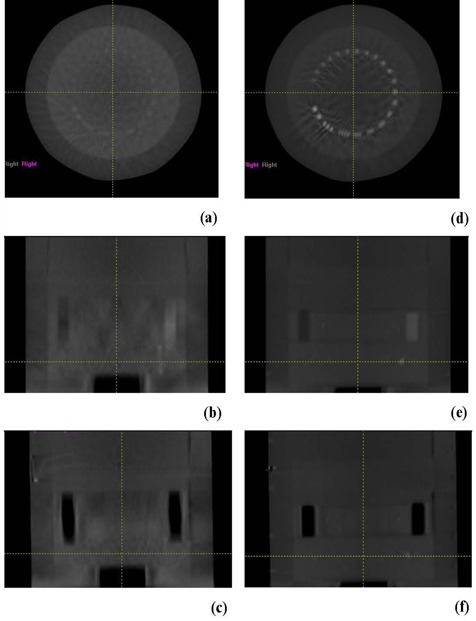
CBCT transverse, coronal and sagittal images, respectively, of the position resolution module of the Catphan reconstructed from cone‐beam projections: before motion correction (a‐c); after motion correction (d‐f).
